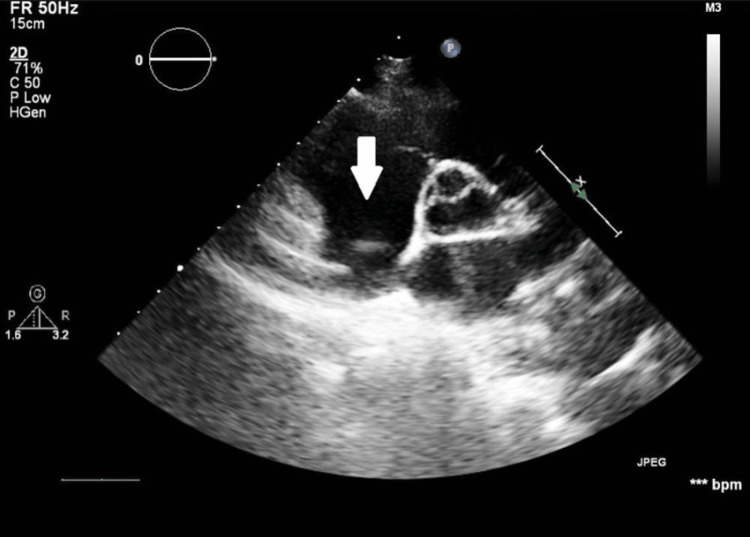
TTE in the short-axis view showing the absence of the previously observed round-shaped mass (white arrow) within the right atrium cavity TTE, transthoracic echocardiogram
